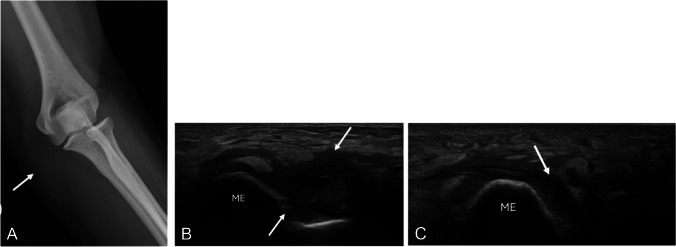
A 16-year-old male presented with medial elbow pain, numbness, and tingling following a football injury. A Radiograph of the elbow demonstrates marked medial soft tissue swelling without evidence of fracture. B Long-axis ultrasound image of the affected elbow reveals a large fluid-filled gap (*between arrows*) extending from the subcutaneous soft tissues to the medial epicondyle, consistent with complete tears of both the common flexor tendon and the ulnar collateral ligament. C Short-axis ultrasound image shows obscuration of the ulnar nerve, which is surrounded by fluid and hematoma. The patient subsequently underwent surgical repair of the common flexor tendon and ulnar collateral ligament reconstruction
